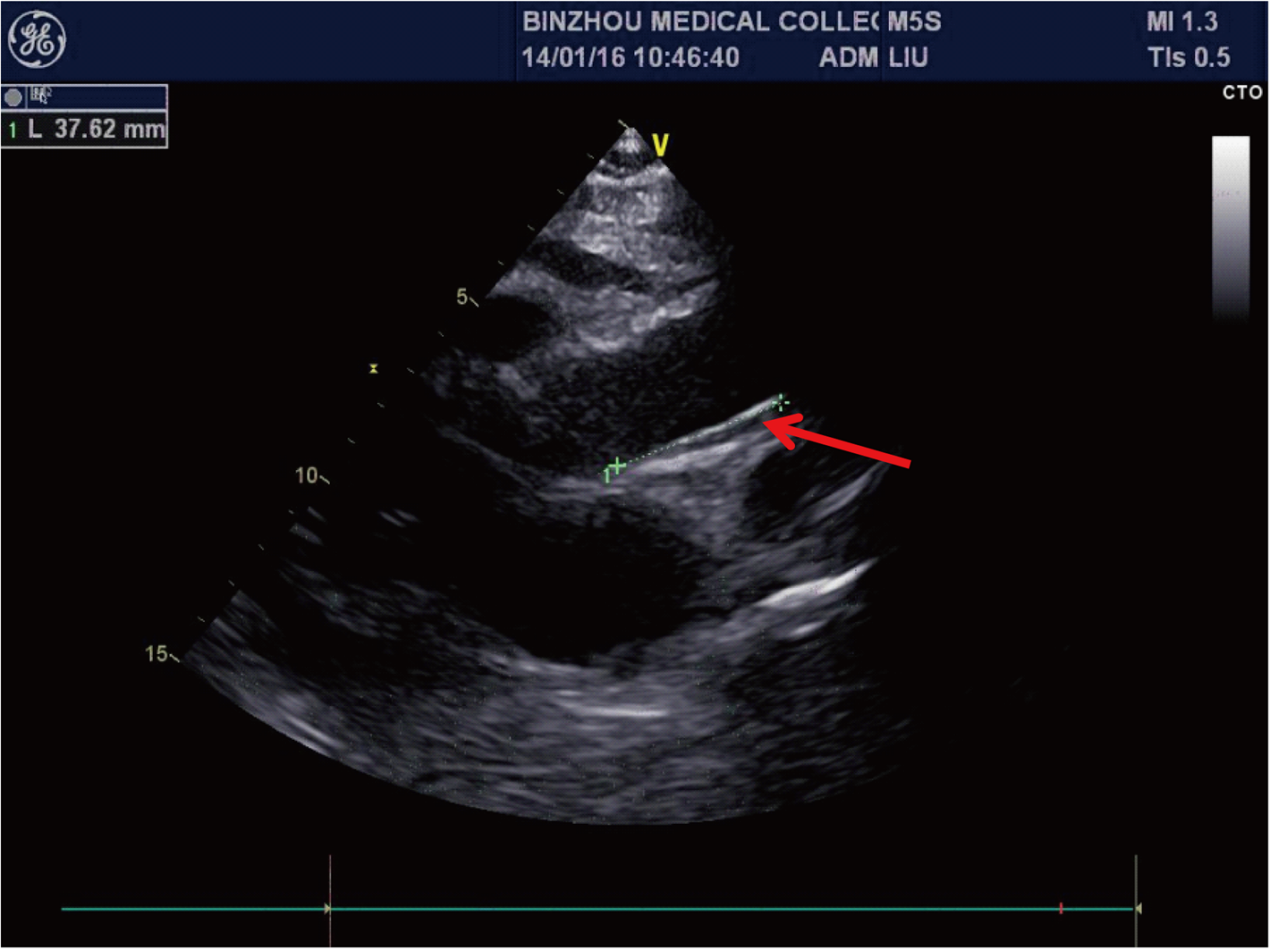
Cardiac colour ultrasound suggesting aortic hematoma or dissection (red arrow)
